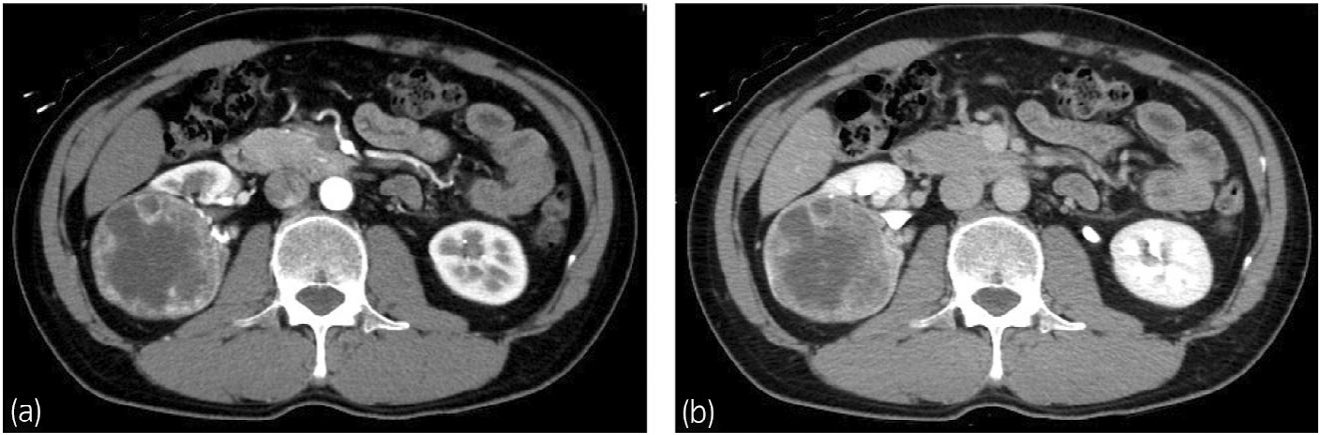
Preoperative computed tomography. A 67‐mm tumor with contrast enhancement was observed in the middle of the right kidney. The tumor is extensively proximal to the urinary tract. (a) Coronal section, arterial phase, (b) Coronal section, excretory phase.
